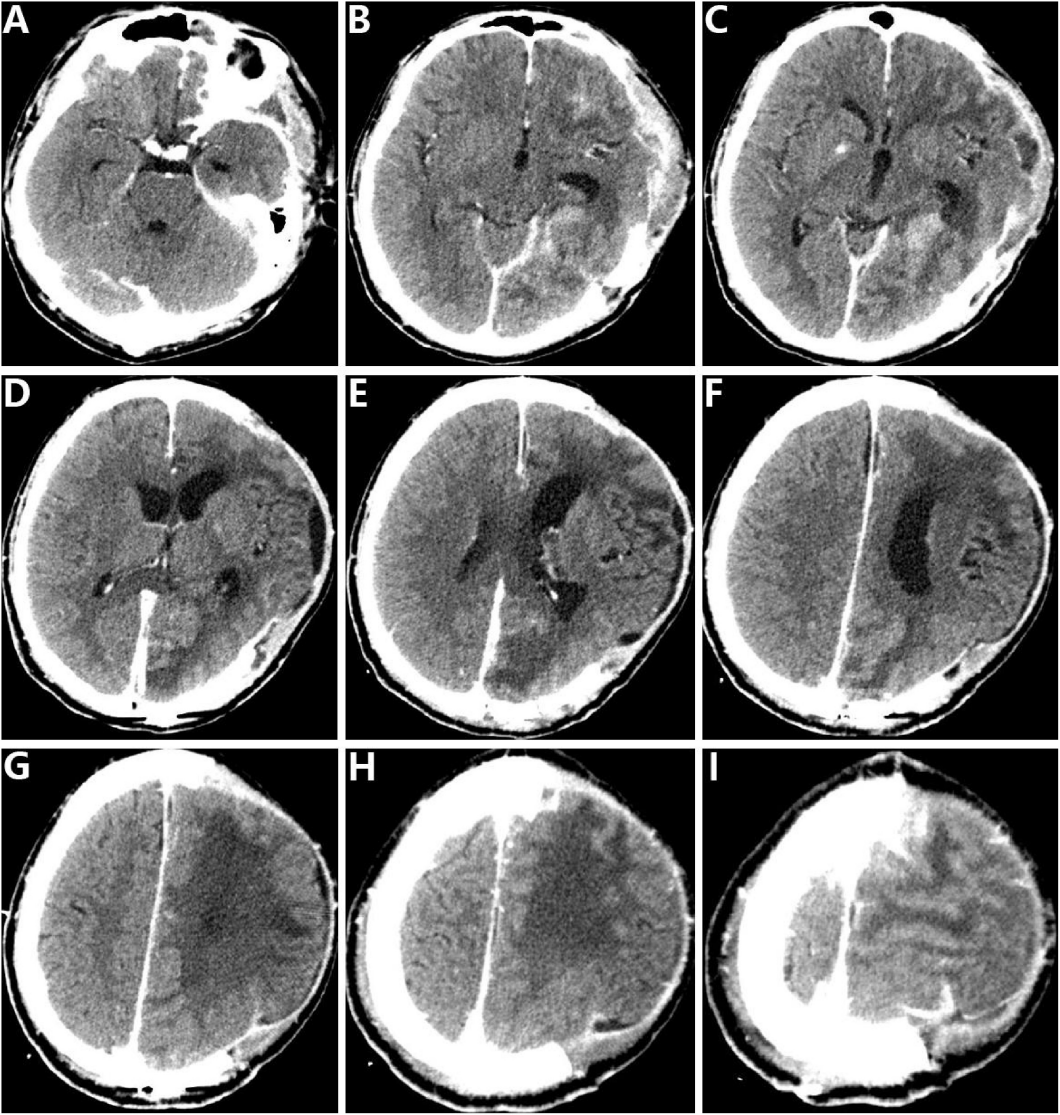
Postoperative head enhanced CT of the patient (postoperative CT). (A–I) Postoperative head CT images of the patient showing the condition of brain abscesses.
